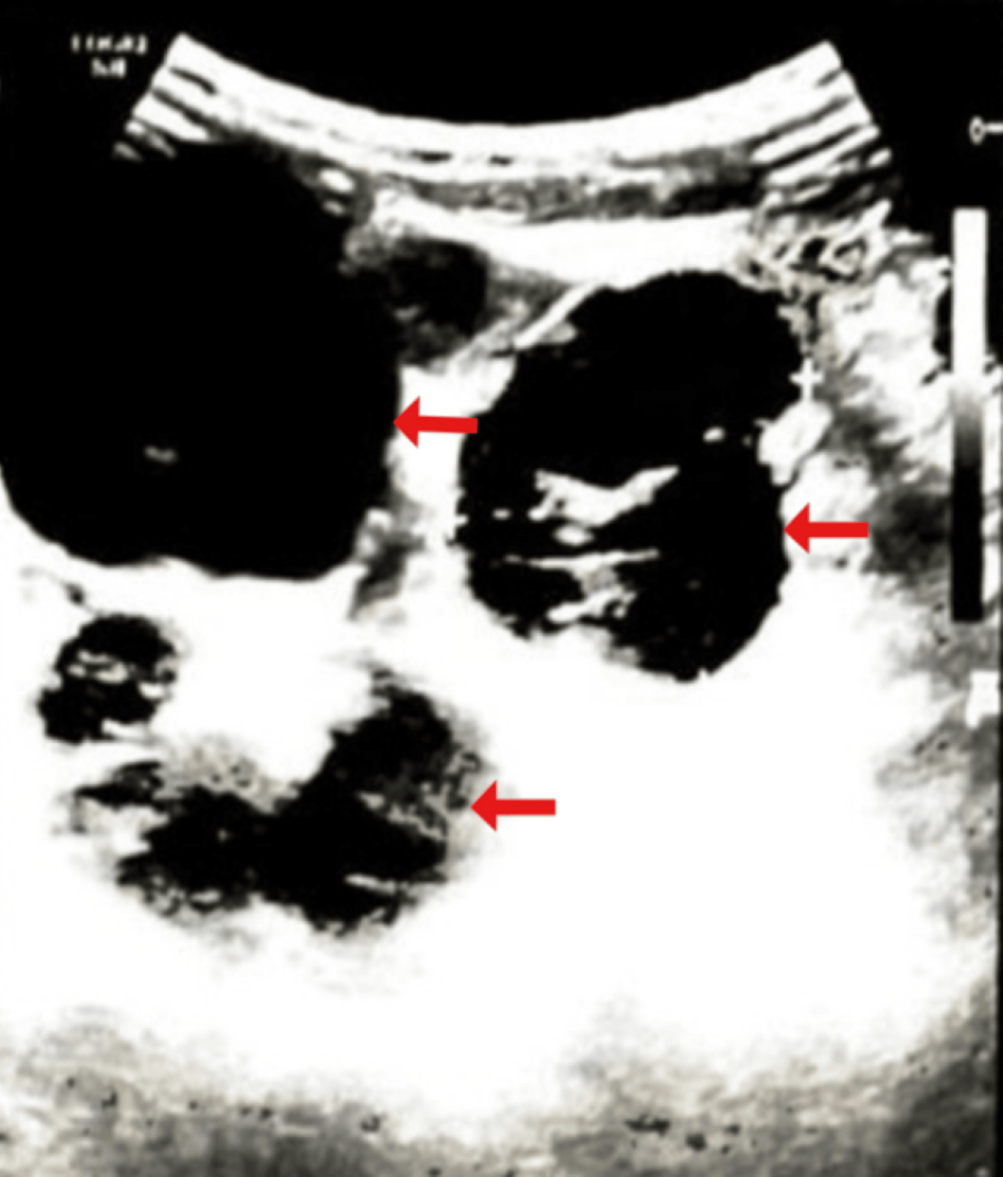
USG showing multiple enlarged round to oval lymph nodes that were found extending from the left side of the aortic bifurcation along the iliac vessels up to the inguinal region USG: ultrasonography
